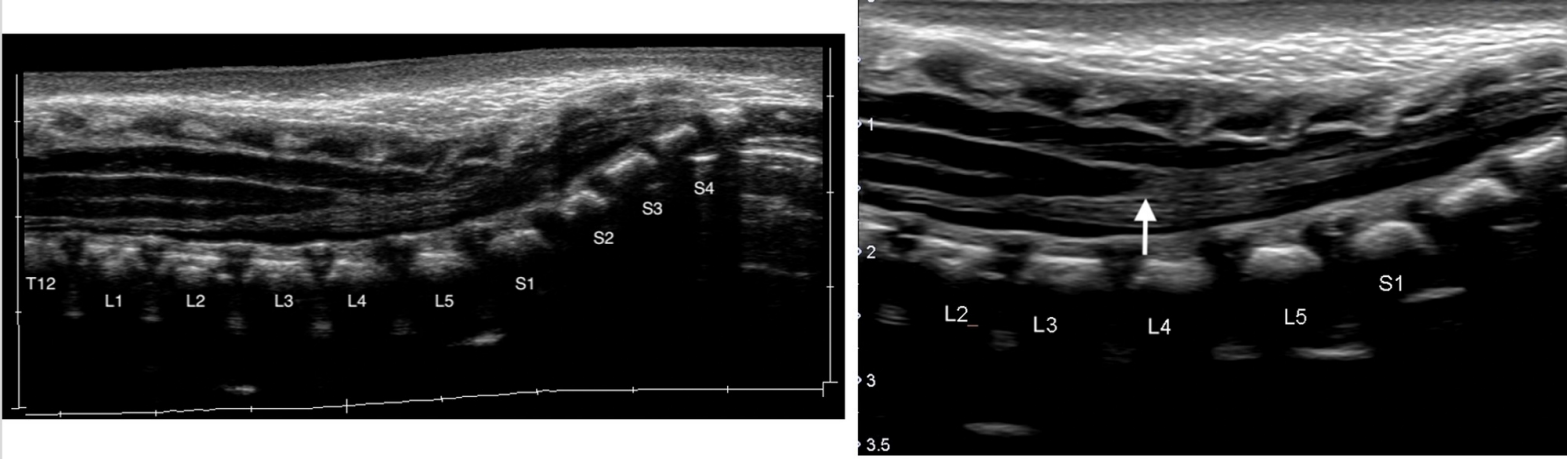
Ultrasound of the lumbar spine showing low-lying conus medullaris at the L4 vertebrae (white arrow)
